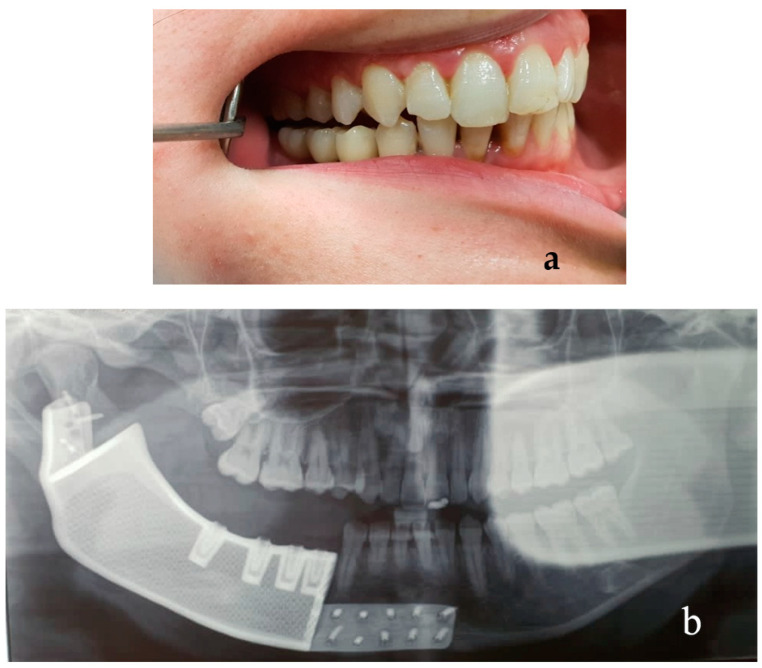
(a) Post-surgery view of intraoral rehabilitation. (b) Post-surgery panoramic X-ray of the positioned mandibular prosthesis.
